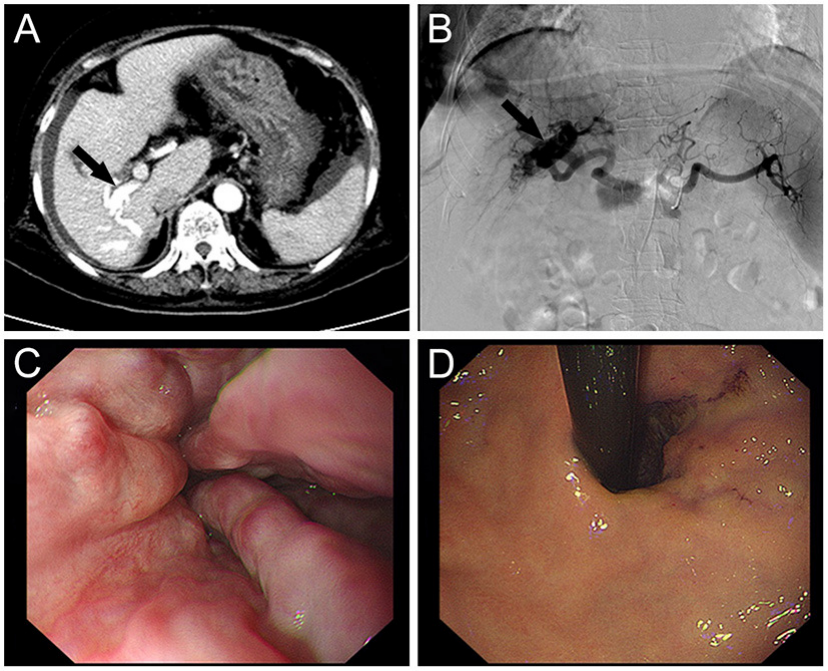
(A) Enhanced CT shows early portal vein visualization in the arterial phase suggesting hepatic arterioportal fistula (black arrow). (B) Selective hepatic artery angiography demonstrated the dilated right hepatic arteries (black arrow). The left hepatic artery was normal. (C) Endoscopy display the tortuous varices with dilatation and positive RC sign in esophagus. (D) Endoscopy display the tortuous varices with dilatation and positive RC sign in gastric fundus.
